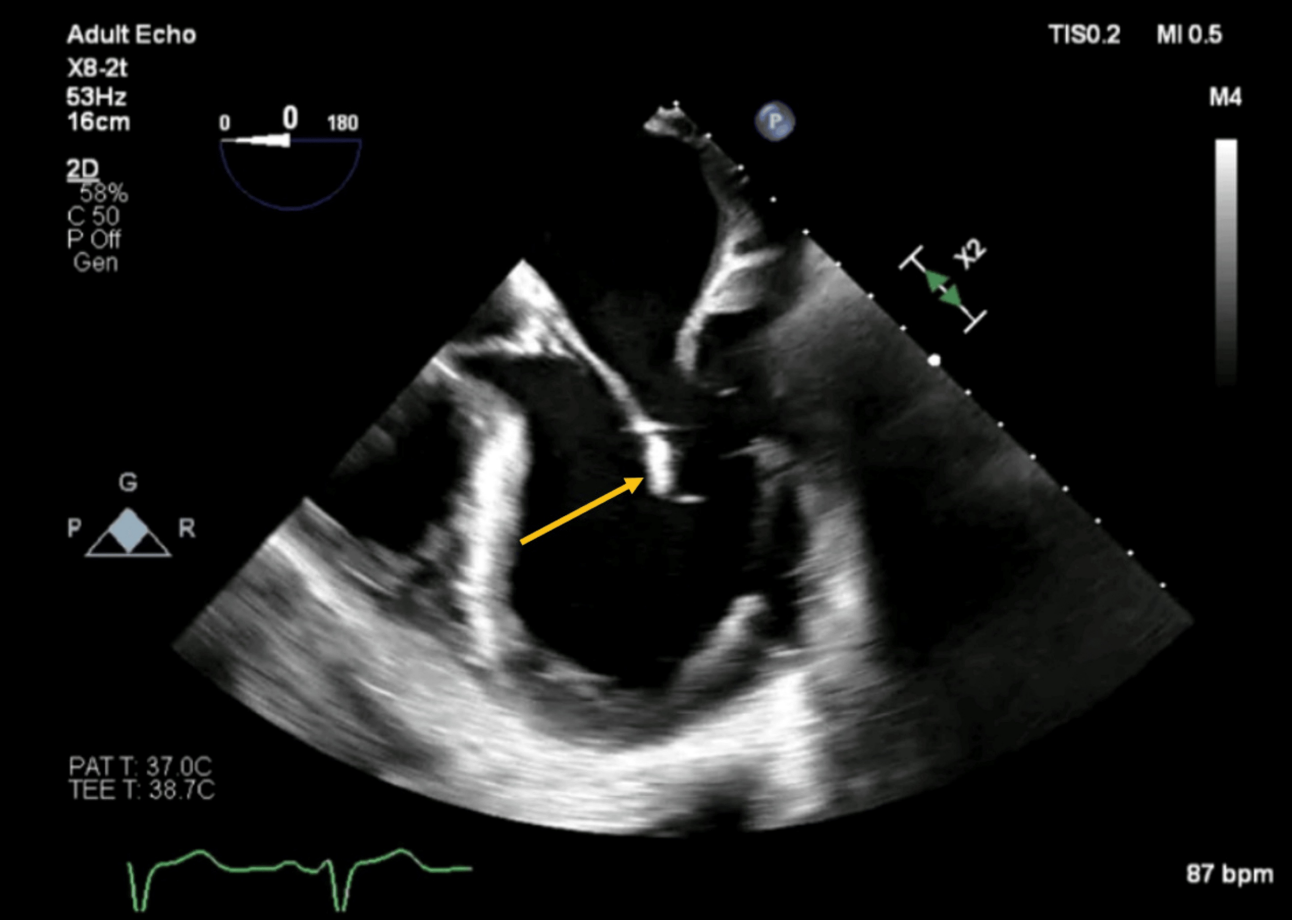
Vegetations in transesophageal echocardiogram
